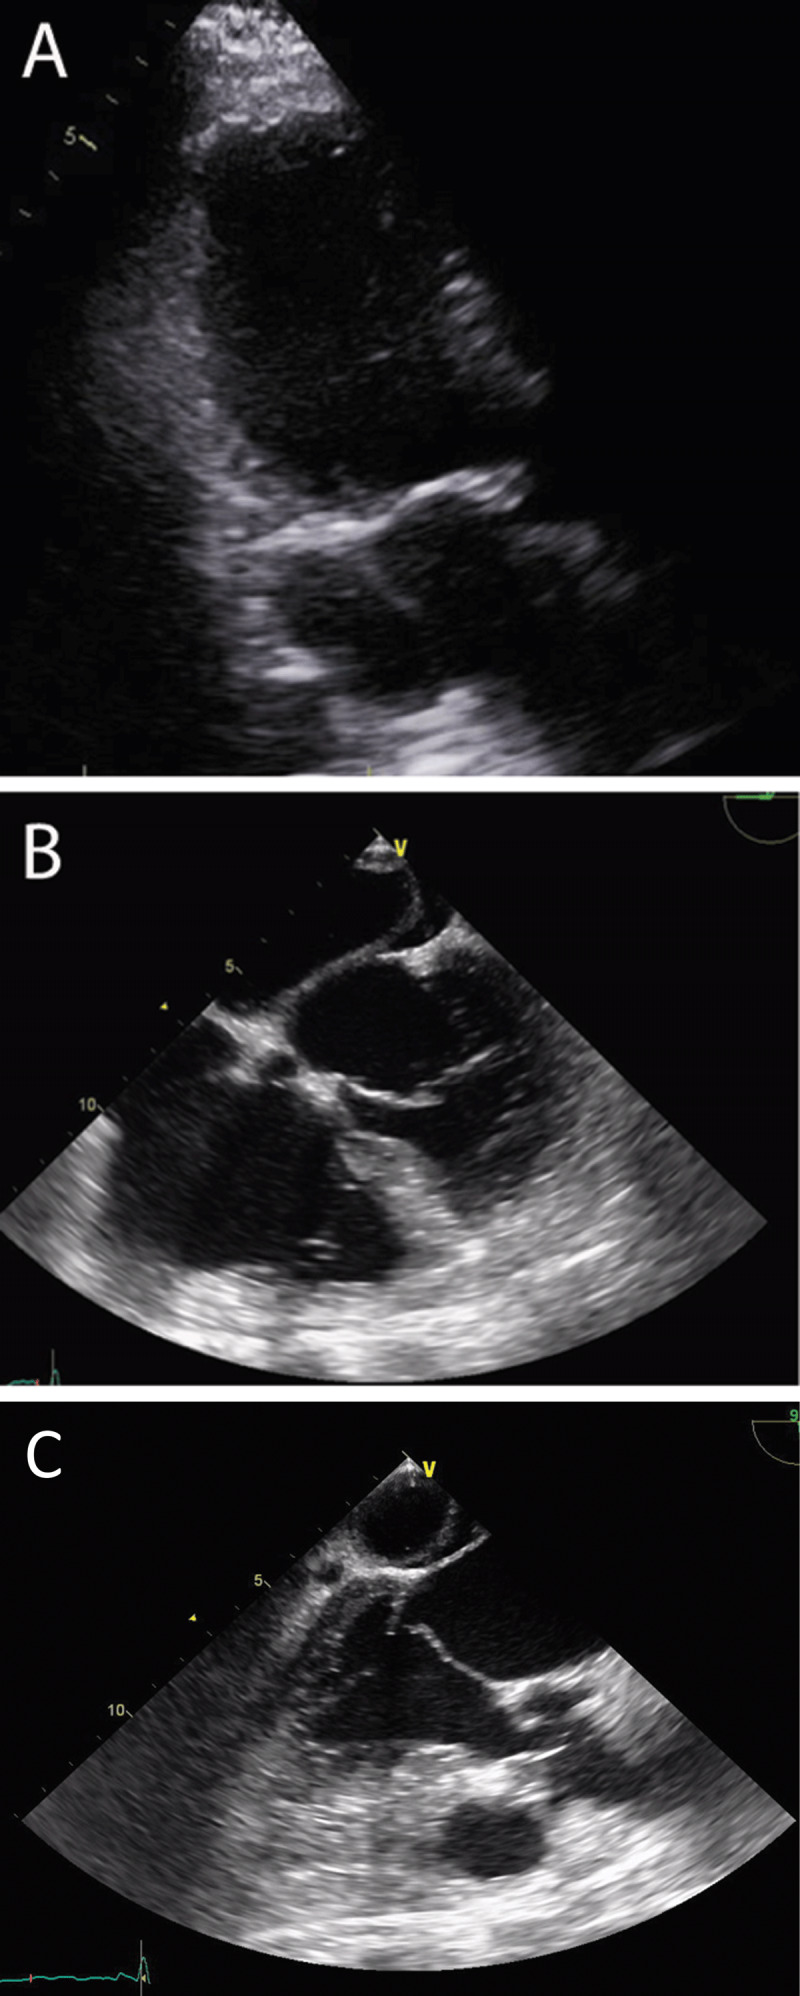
(A) Three-chamber view on transthoracic echocardiogram shows the cystic structure with no contrast flow after normal saline injection. (B) Four-chamber view on transesophageal echocardiogram showing the cystic structure in relation to the left atrium. (C) Tethered posterior leaflet.
